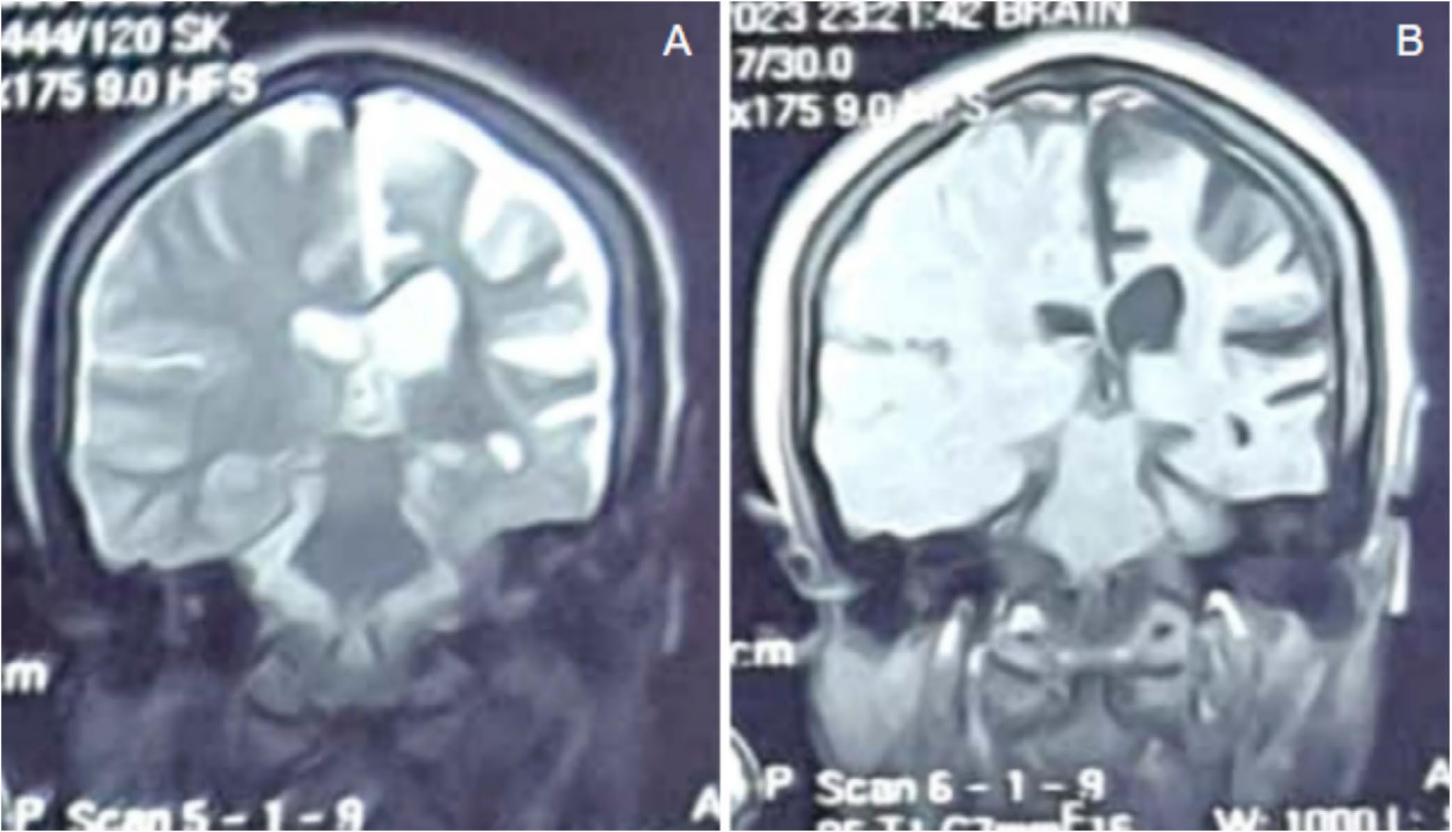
(A) T2 coronal MRI showing hyperpneumatization of left mastoid air cells with slight elevation of the left petrous ridge. (B) T1 post‐contrast coronal MRI demonstrating left lateral ventricle dilation and calvarial thickening.
